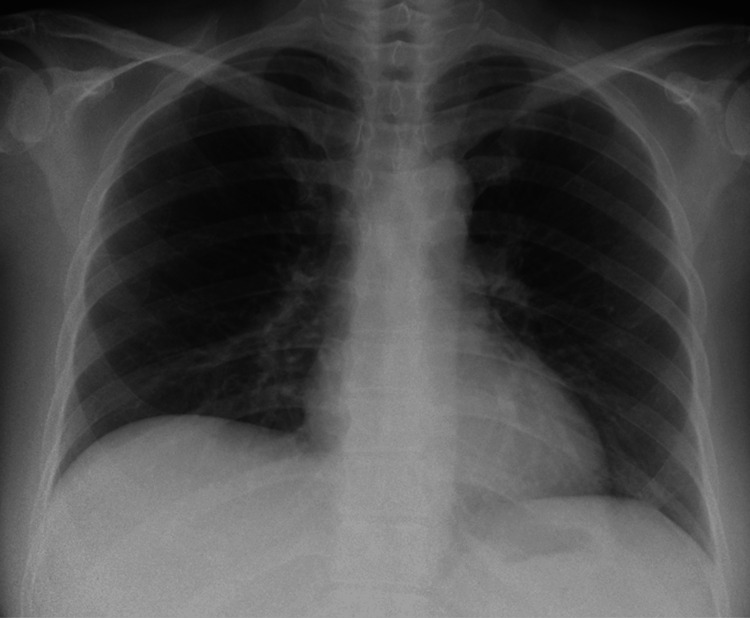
Chest radiograph of the first case No significant findings were evident.
